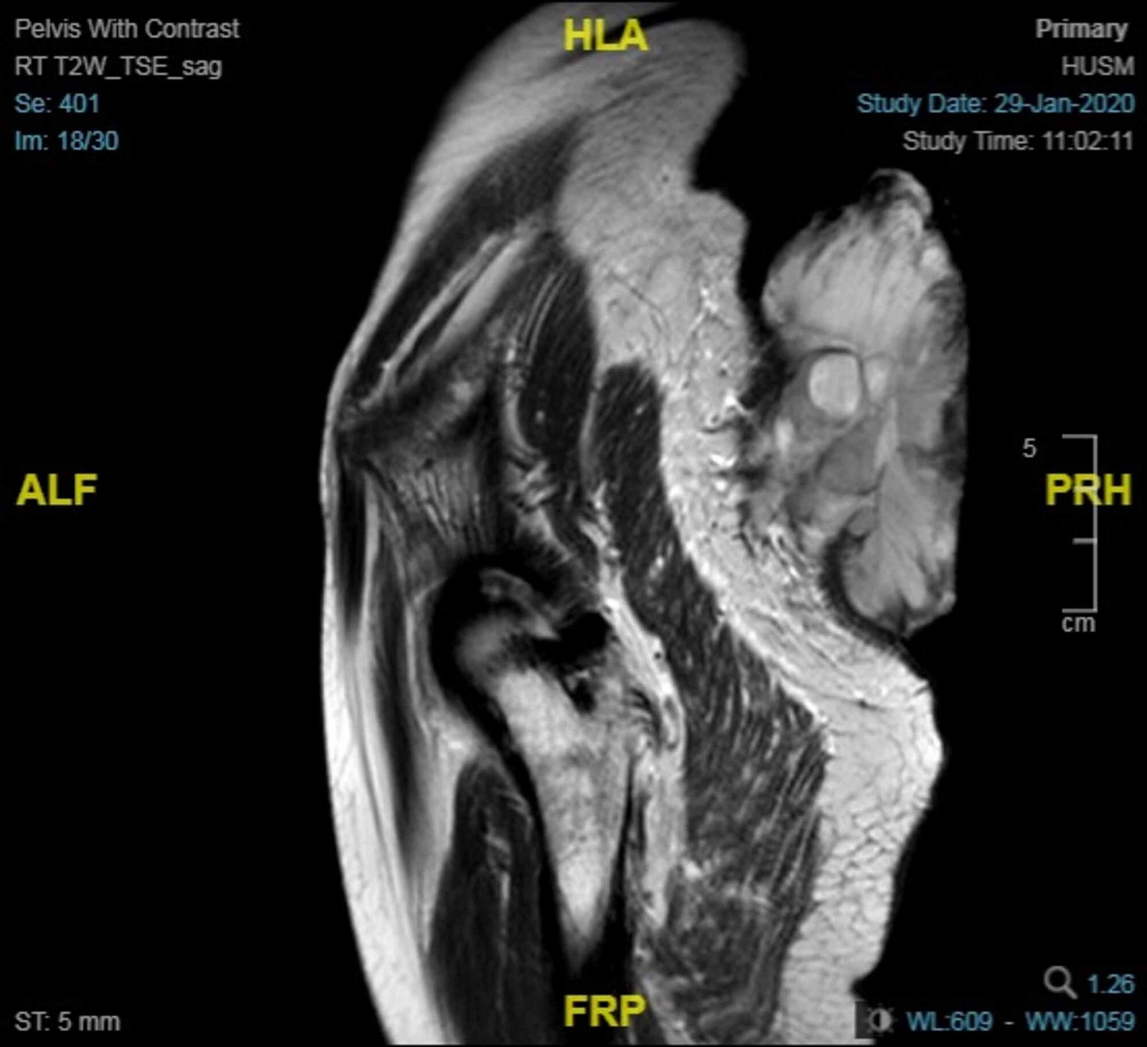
Longitudinal section MRI showing cutaneous pedunculated exophytic mass at the posterior pelvic region with subcutaneous fascia involvement.
